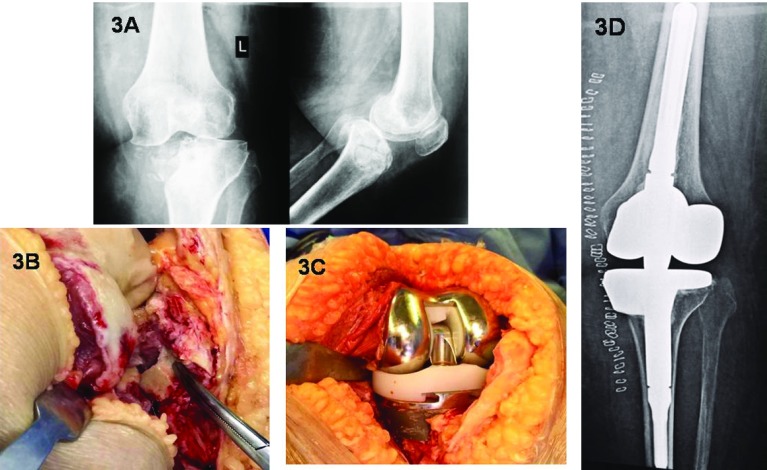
(A) Preoperative X-rays for a depressed fracture of the medial tibial plateau. Metaphyseal collapse and knee subluxation can be observed. (B) Intraoperative photo shows unreconstructable comminution of the articular surface. (C) The bone defect was reconstructed using a medial 20 mm metal step attached to the tibial tray. (D) Postoperative X-ray showing the Rotating Hinge implant and metal augment to the proximal tibia.
